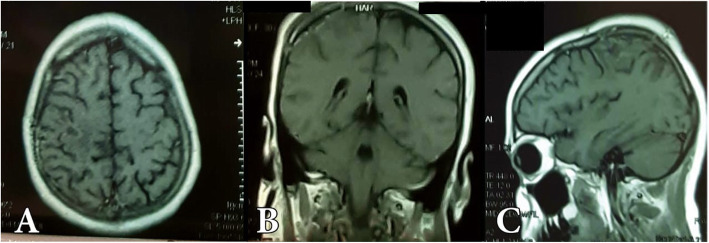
Postoperative MRI with gadolinium of the same patient in axial (A), coronal (B), and sagittal (C) projections showing total tumor resection
